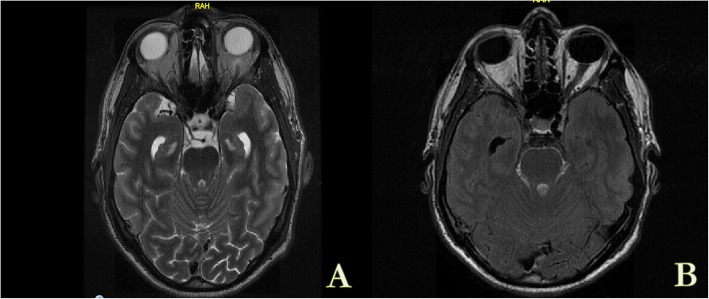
MRI Brain with diffuse smooth, leptomeningeal enhancement, predominantly within the basal cisterns and surrounding brainstem associated with cranial nerve enhancement most pronounced along the pre-chiasmatic optic nerves and 3rd cranial nerves with interval development of hydrocephalus noted. a Axial T2 Flair. b Axial T1 Flair post Gadolinium enhancement
